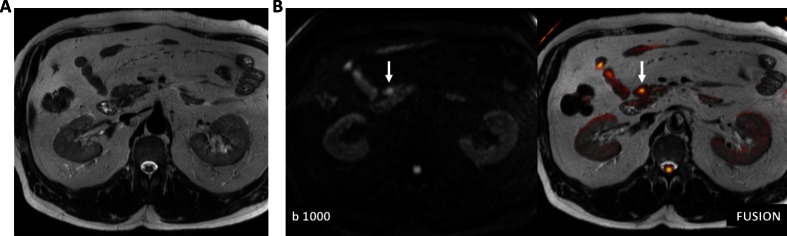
Diffusion-weighted MRI (DW-MRI) of the pancreas. a Axial HASTE T2-weighted image does not depict any abnormality in the uncinate process of the pancreas. b DW image (*left*) at high *b* value (*b* = 1000 s/mm^2^) and fused image (*right*) superimposing axial T2-weighted MRI image and color-coded map derived from high *b* value (*b* = 1000 s/mm^2^) DW image clearly demonstrate a small pancreatic neuroendocrine tumor (*arrows*) with restricted diffusion at this level
